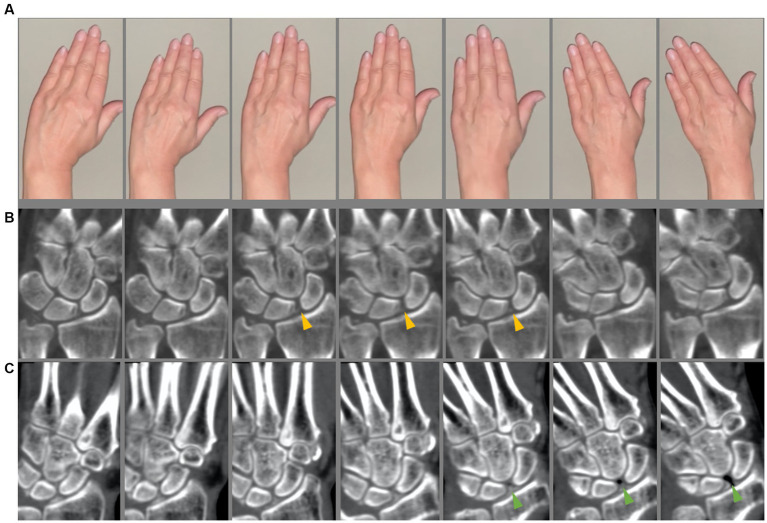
4D-CT scan. (A) Sequences of the instruction video. (B,C) Four-dimensional computed tomography scan in two different calcium pyrophosphate deposition disease (CPPD) patients. (A,B) Slight scapho-lunate (SL) dissociation is seen (yellow arrow heads) indicating a dynamic SL ligament instability. (C) CPPD patient with clearly SL dissociation with vacuum effect (green arrow heads).
